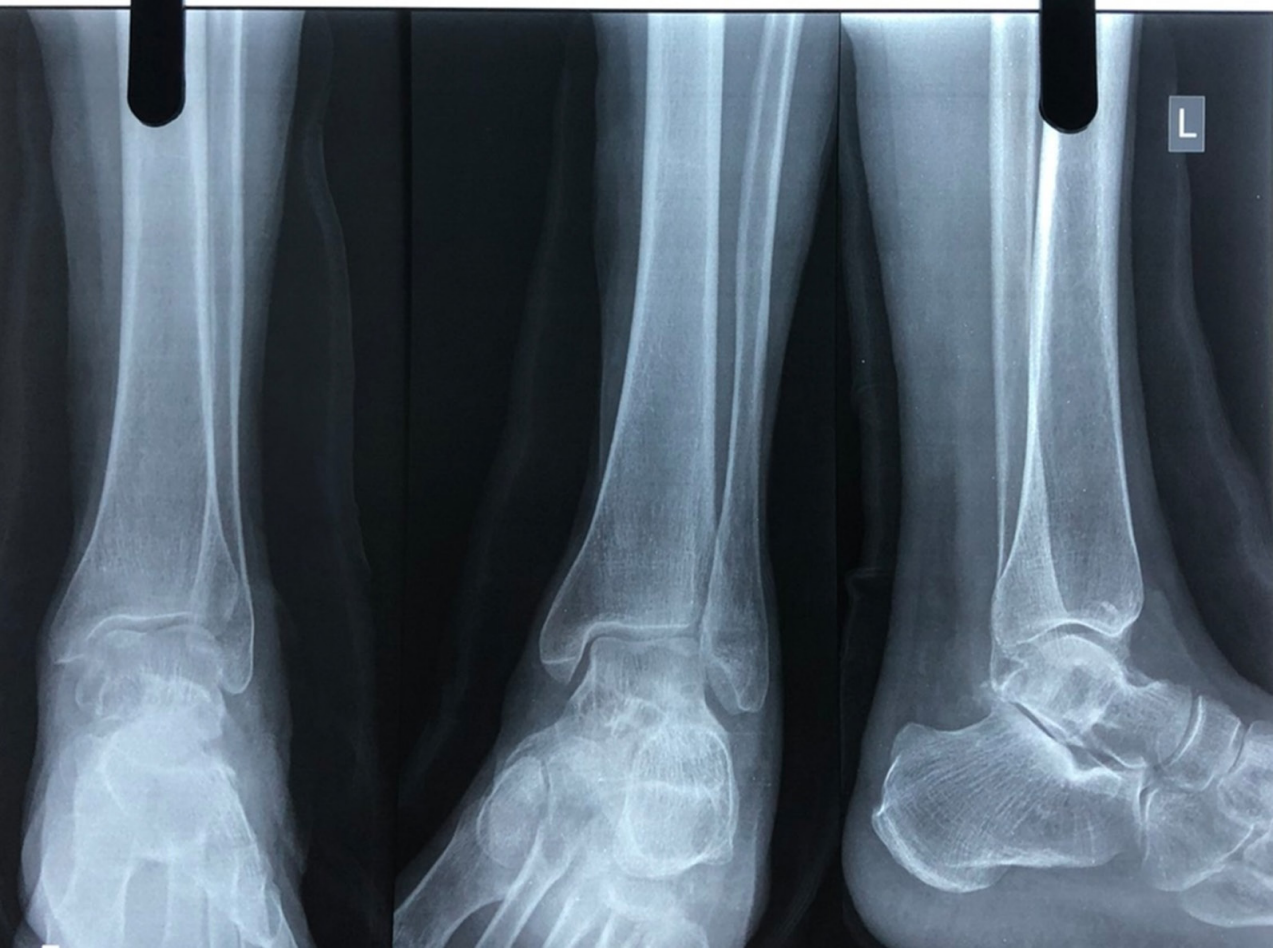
Postoperative radiographs showing complete excision of mass
